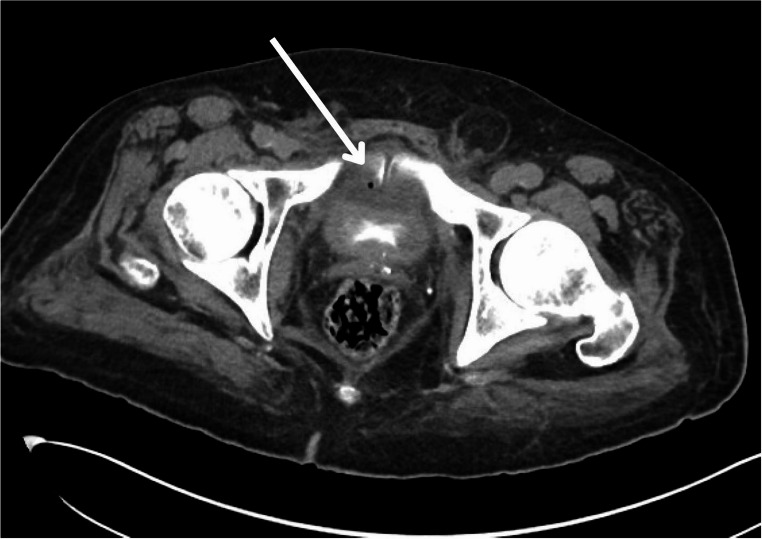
CT Urogram with air adjacent to bladder at the level of the pubic symphysis- an indicator of potential infection and fistula
